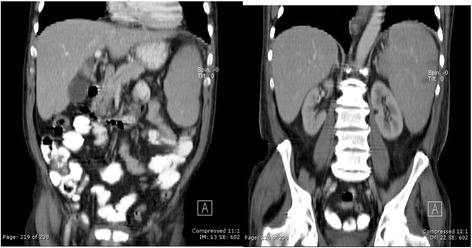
Computed tomography of the abdomen with contrast
